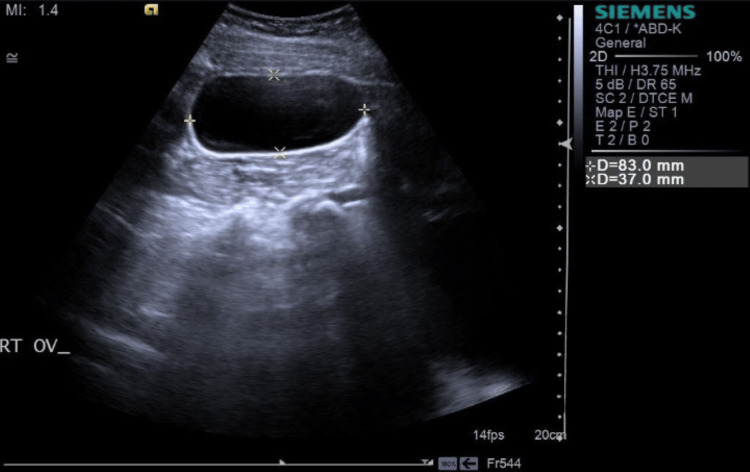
Pelvic ultrasonography showing a right ovary cyst with the dimension of 83.0 mm × 37.0 mm
